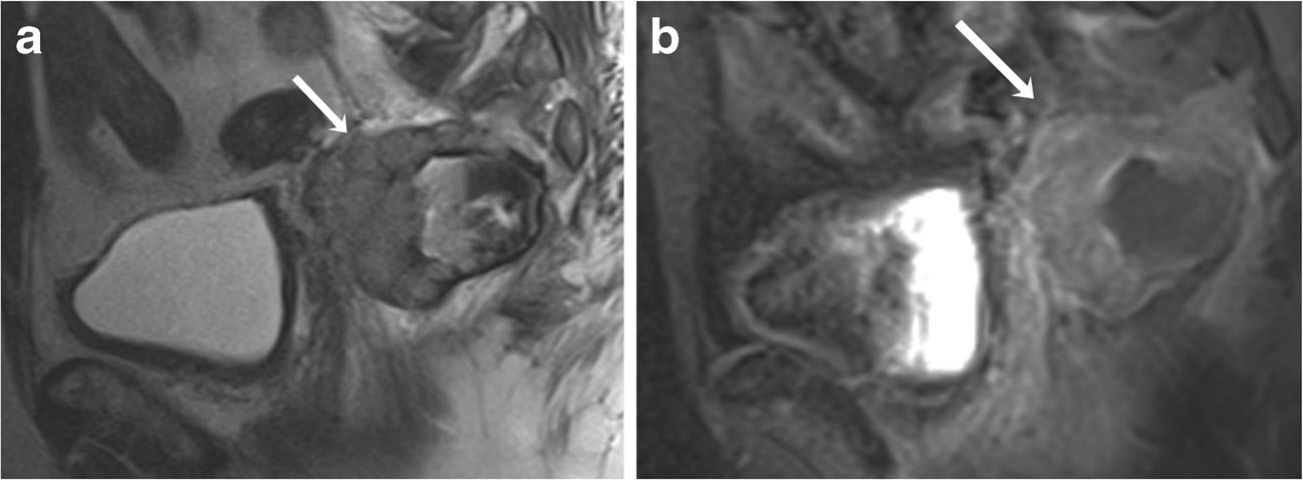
A 76-year-old male with anorectal melanoma. a Sagittal T2-weighted MR image shows a heterogeneous mass lesion arising from the anterior wall of the rectum (arrow). b The mass shows enhancement on the corresponding sagittal fat-suppressed T1-weighted MR image (arrow)
